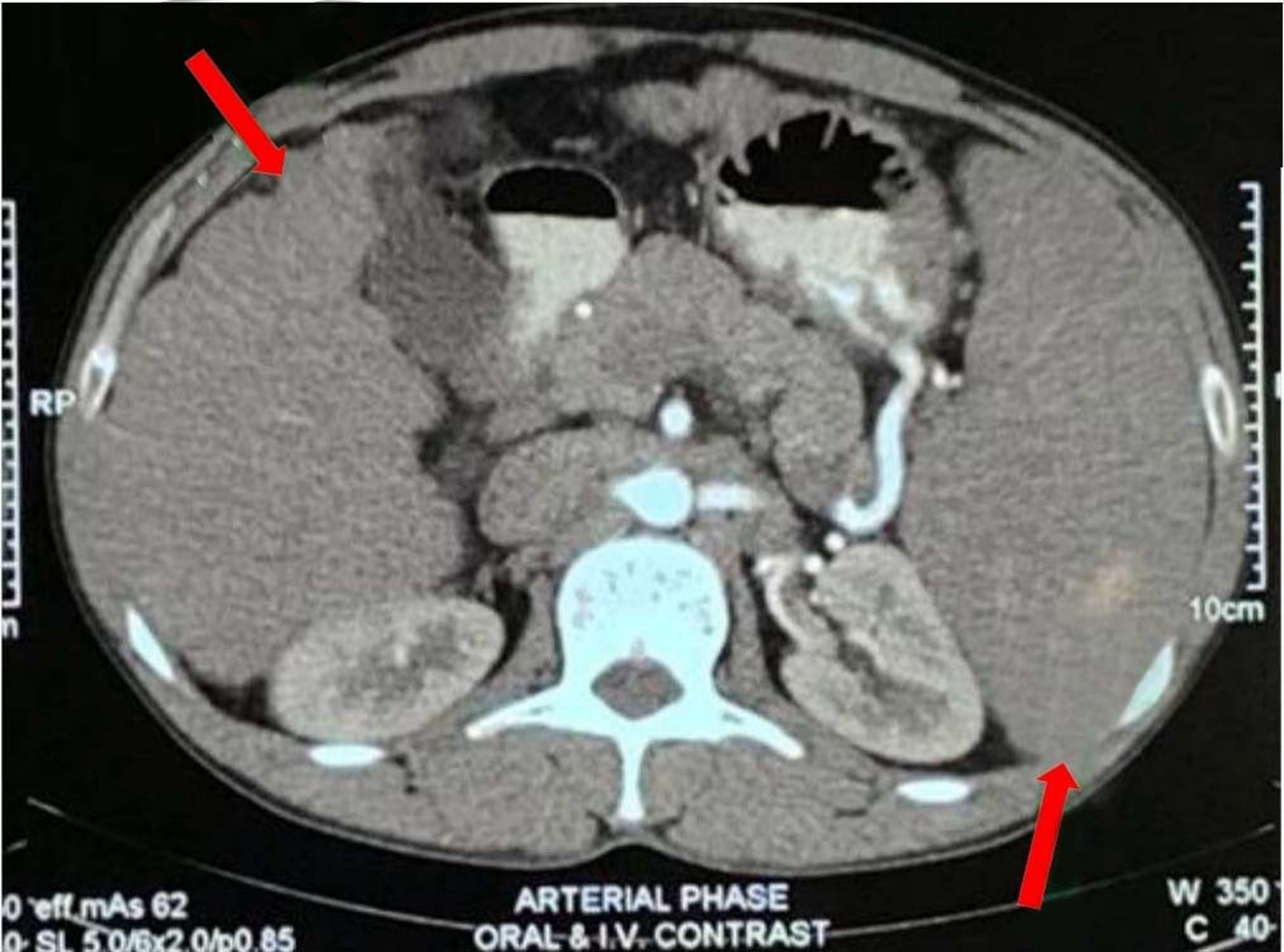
CT scan of abdomen showing nodular liver surface with heterogenous parenchymal attenuation and splenomegaly with nonspecific splenic nodule.
